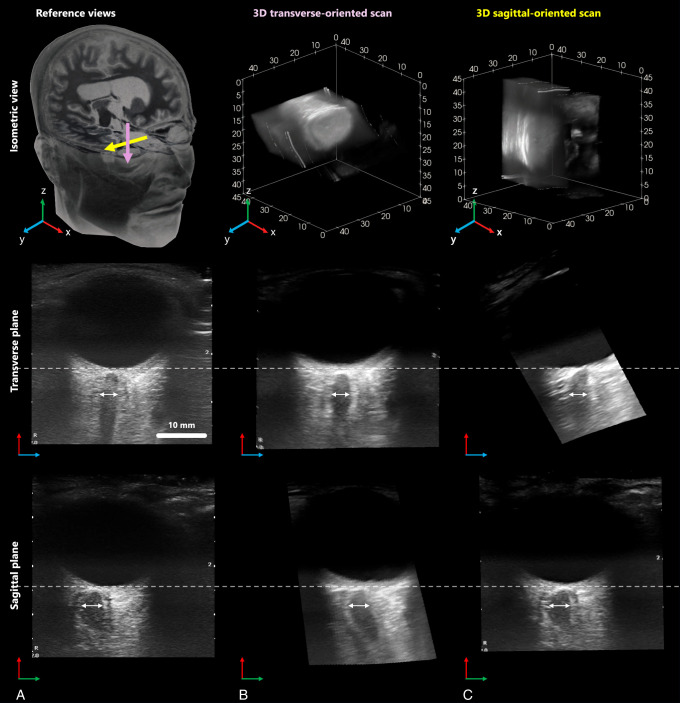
Three-dimensional freehand ultrasound scans of the optic nerve sheath. Column (A) shows the reference scan, column (B) the transversely oriented scan acquired along the *z*-axis (forehead to occiput), and column (C) the sagittally oriented scan acquired along the *y*-axis (nose to temporal region). The top row displays isometric views of the reconstructed 3D volumes, the middle row shows transverse slices at the central plane, and the bottom row shows sagittal slices. White arrows of equal length indicate the spatial comparability across scan orientations. Images have been rotated for visualization purposes.
